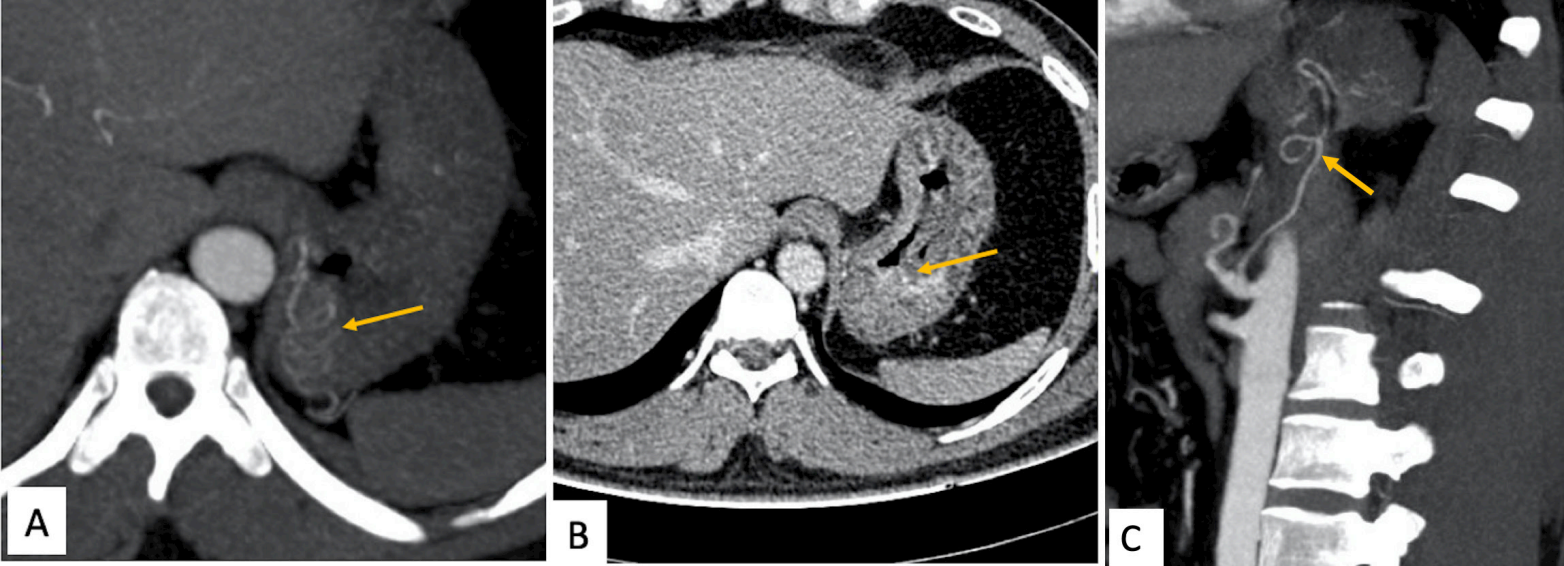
Arterial phase of contrast CT (A and C) showing abnormal
